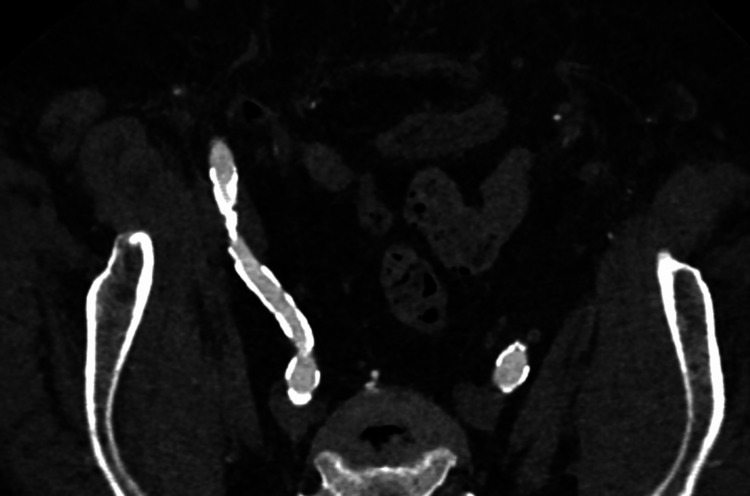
CT angiography demonstrating bilaterally heavily calcified EIA. CT, computed tomography; EIA, external iliac artery
